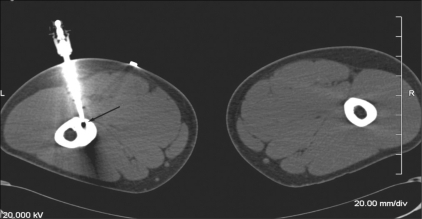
Osteoid osteoma of the femur. CT scan in the supine position shows the position of the electrode (arrow) within the nidus
